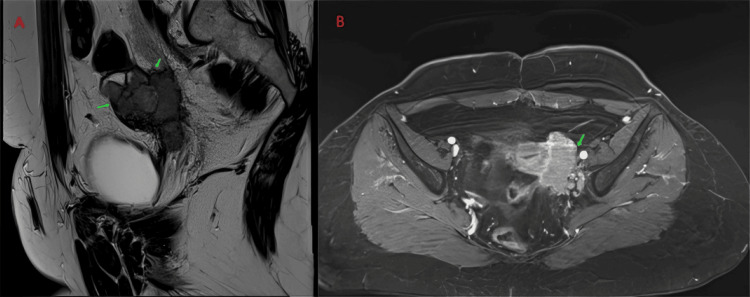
Pelvic MRI showing the presence of a solid-cystic mass in the left ovary (green arrows) (A) Sagittal section. (B) Axial section.
